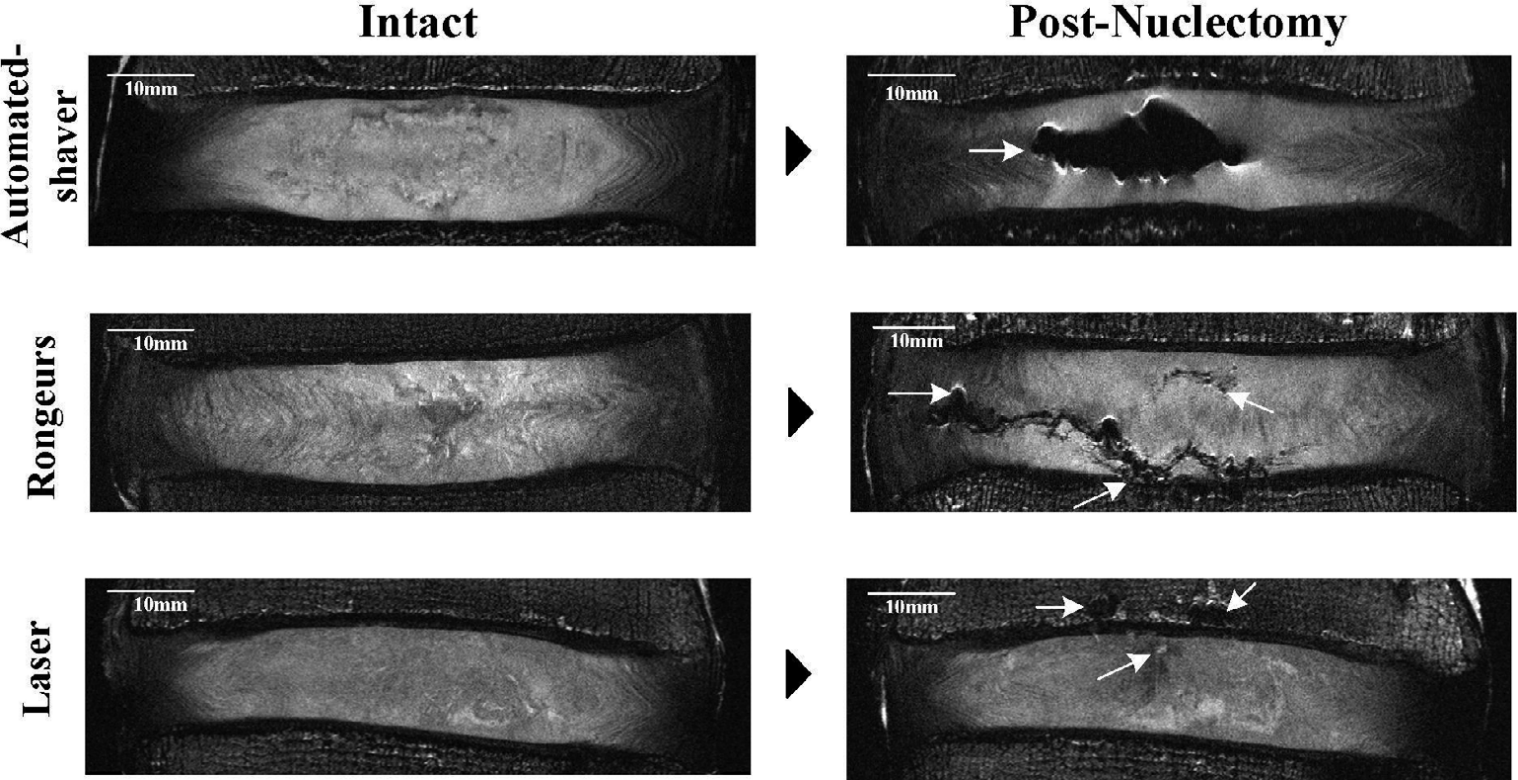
Typical coronal plane T2 weighted MRI of discs at both the intact and post‐nuclectomy stages. The white arrows indicate locations of damage. Using the automated‐shaver disc material was removed homogeneously approximately at the center of the disc (top row). Using rongeurs disc material was removed nonhomogeneously with some damage seen in the AF and at the endplates (middle row), and finally using laser, cavities were formed in the NP region that may have filled with condensed vapor, and cavitations were seen in the subchondral bone marrow (bottom row).
